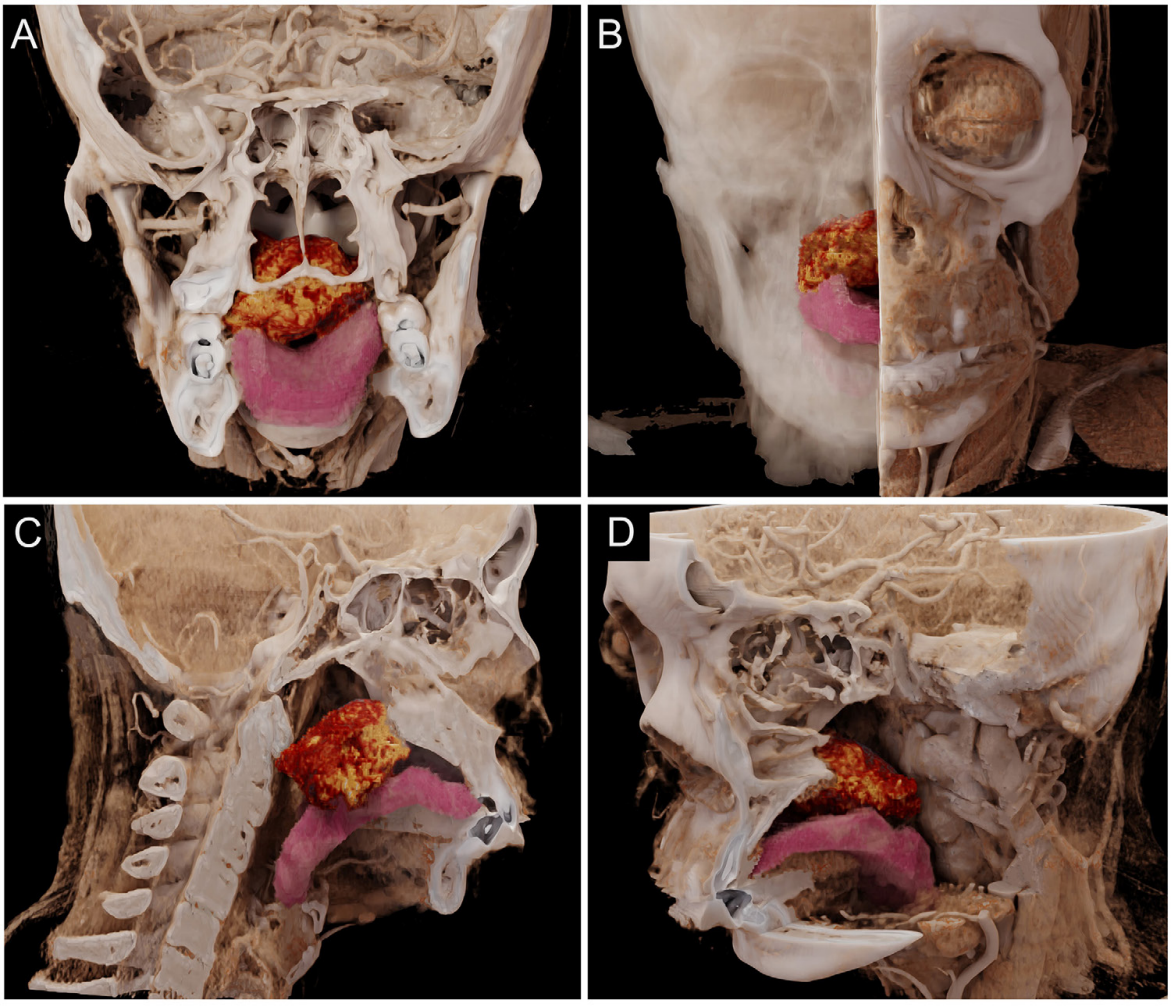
Hyper‑realistic rendering (HRR) of CT for palatal myoepithelioma.
